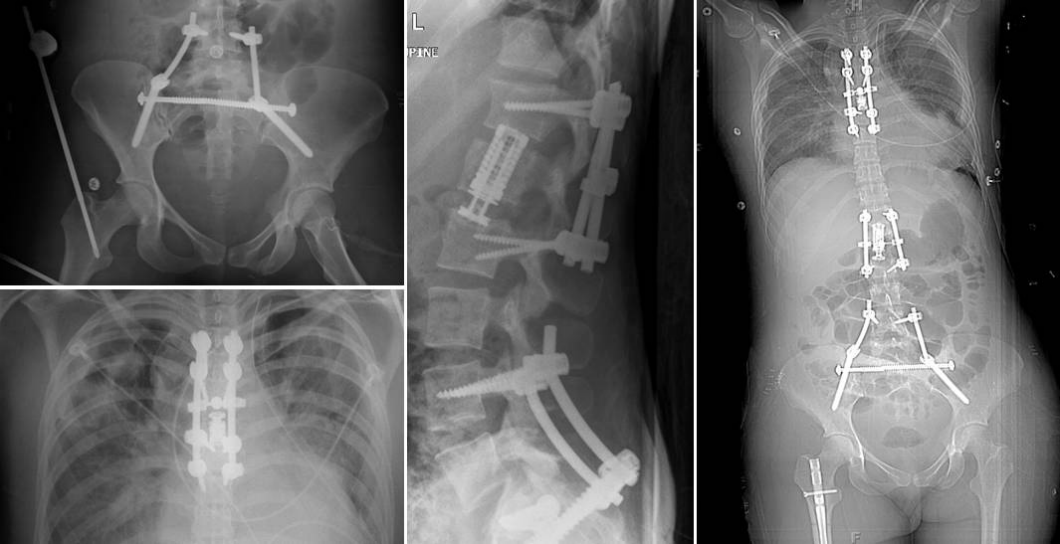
Postoperative X-rays after stabilization of the pelvic ring injury with bilateral lumbo-pelvic/triangular osteosynthesis, and 360° fusion of the unstable T6 and L1 injuries.
